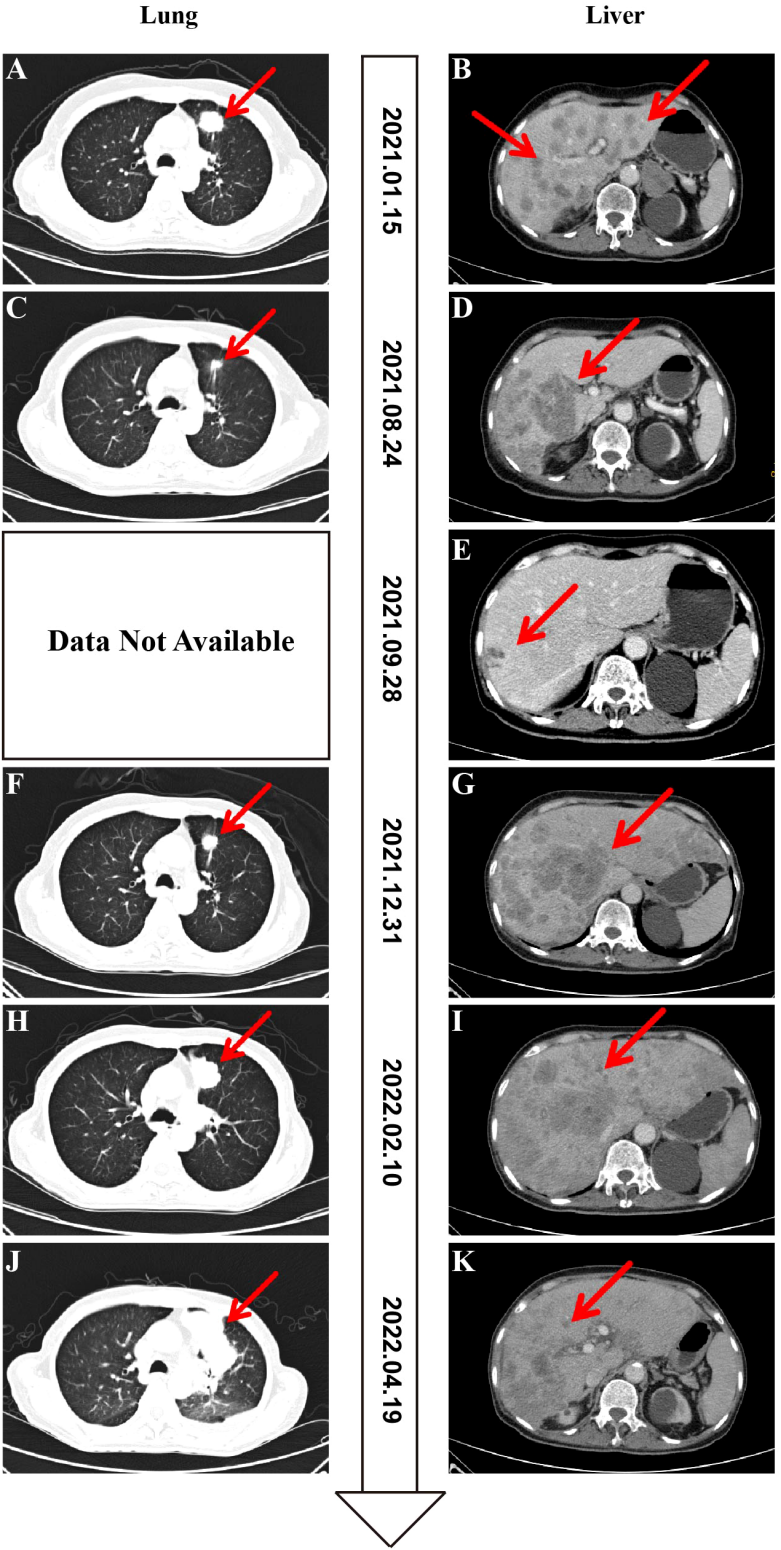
Computed tomography (CT) of this patient at diagnosis and follow-up. (A, B) Baseline CT at diagnosis of the lung (A) and liver (B). (C, D) CT after 7.5 months of osimertinib therapy with decreased size of the left lung (C) and progressive disease of the liver (D). (E) CT after around 1 month of osimertinib plus pralsetinib therapy with decreased size and number of liver metastases. (F, G) CT after 4 months of osimertinib plus pralsetinib therapy with progressive disease of the left lung (F) and liver (G). (H, I) CT after 1 month of alectinib therapy with progressive disease of the left lung (H) and stable disease of the liver (I). (J, K) CT after 3 months of alectinib plus osimertinib therapy with progressive disease of the left lung (J) and stable disease of the liver (K).
